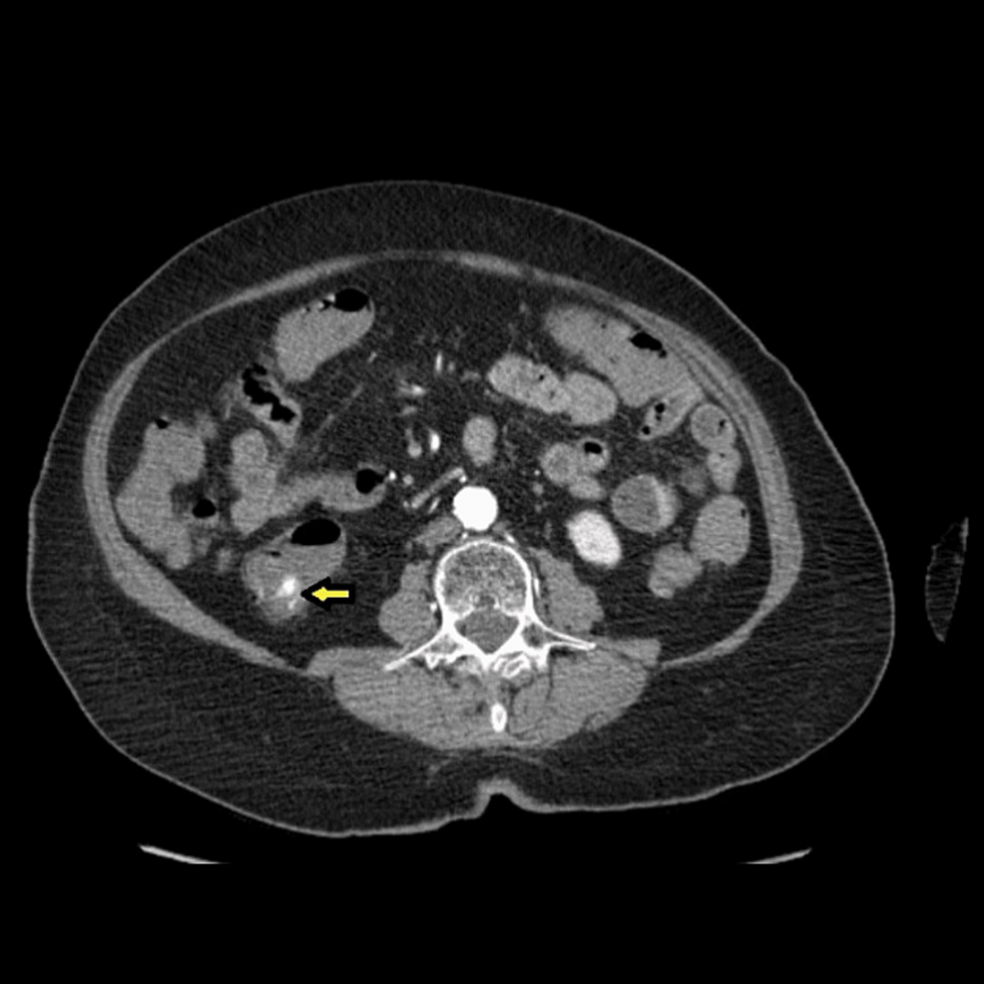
CT of the abdomen showing active bleeding in the proximal ascending colon (yellow arrow)
